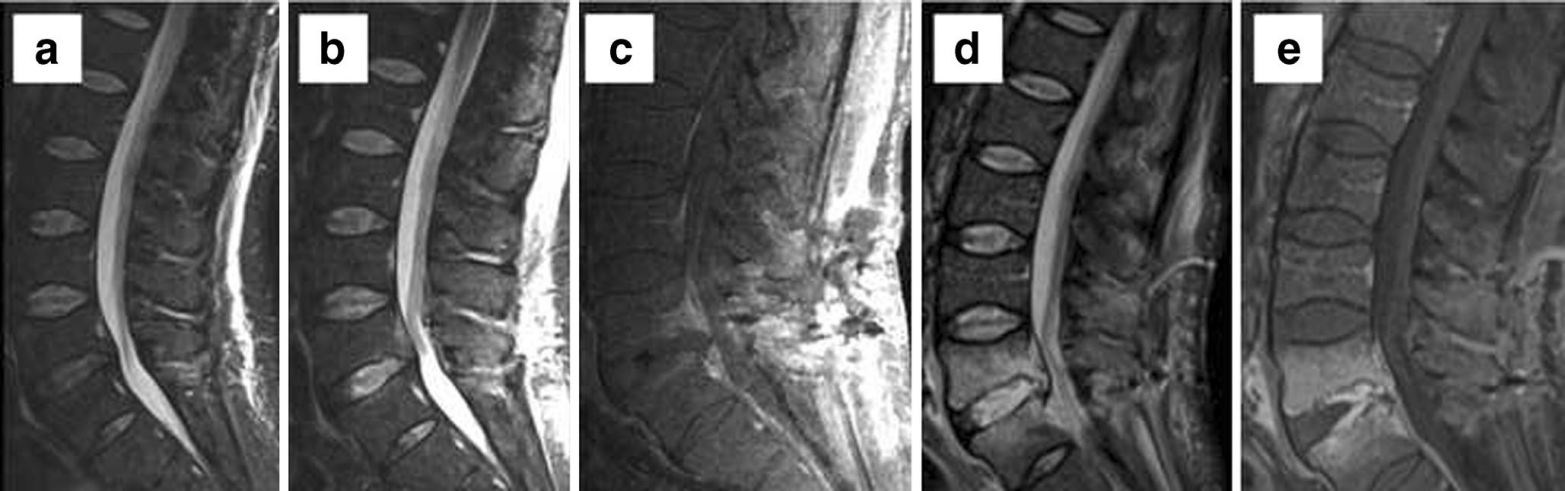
Evolution of changes over time post discectomy at L4–5. Sagittal STIR image (a) shows minimally increased T2 signal in the disc posteriorly and preserved marrow signal. Two-week follow-up MRI shows interval increased signal through most of the disc (b) with marrow oedema and enhancement on postcontrast images (c). Significant interval worsening was seen on further follow-up on both STIR (d) and postcontrast images (e) with epidural phlegmon
